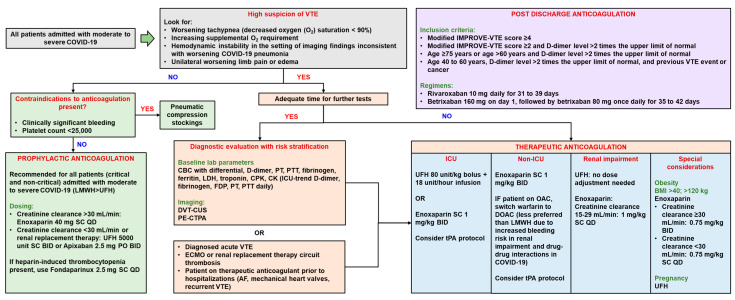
Proposed workflow of the management of COVID-19-associated thromboembolism by Hajra et al. [78]. (AF = atrial fibrillation, BID = twice a day, BMI = body mass index, CBC = complete blood count, CK = creatinine kinase, CPK = creatinine phosphokinase, CTPA = computed tomography pulmonary angiography, CUS = compression ultrasonography, DOAC = direct oral anticoagulant, DVT = deep-vein thrombosis, ECMO = extracorporeal membrane oxygenation, ICU = intensive care unit, IMPROVE = International Medical Prevention Registry on Venous Thromboembolism, LDH = lactate dehydrogenase, LMWH = low-molecular-weight heparin, OAC = oral anticoagulation, PE = pulmonary embolism, PT = prothrombin time, PTT = partial thromboplastin time, QD = once a day, SC = subcutaneous, tPA = tissue plasminogen activator, UFH = unfractionated heparin, and VTE = venous thromboembolism).
